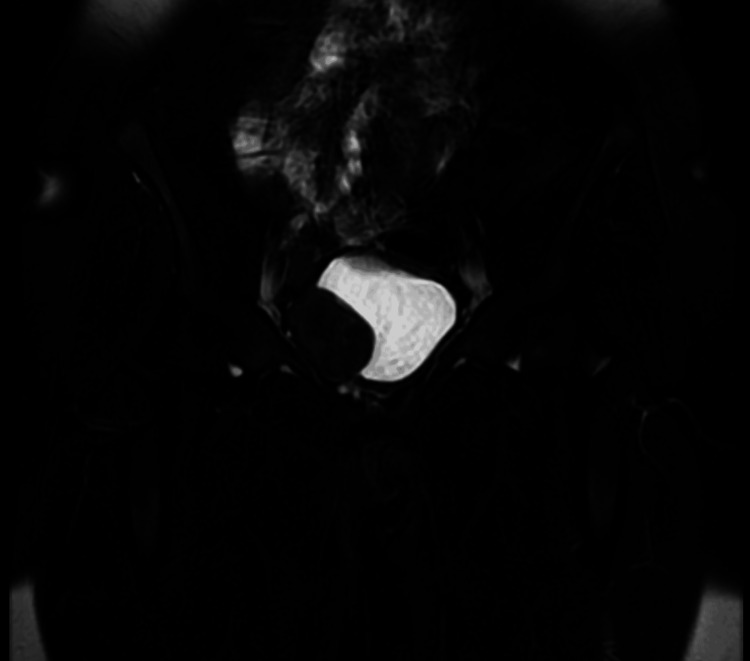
Pre-operative contrast-enhanced pelvic MRI T2 coronal
